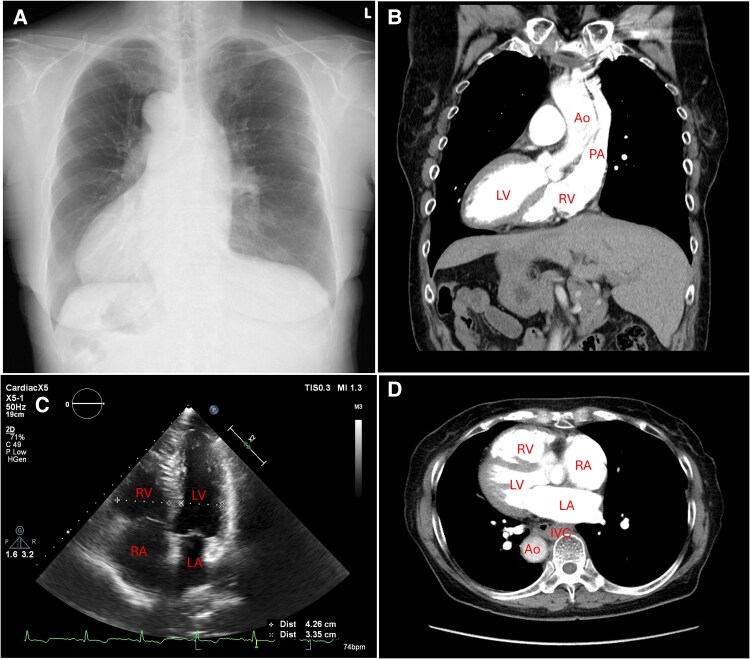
Complete situs inversus on chest X-ray, thoracic echocardiography, and computed tomography. (*A*) Pre-treatment chest X-ray, frontal view. (*B*) Pre-treatment chest computed tomography, coronal view. (*C*) Pre-treatment *trans*-thoracic echocardiogram, four-chamber view. (*D*) Pre-treatment chest computed tomography, axial view. Ao, aorta; IVC, inferior vena cava; LA, left atrium; LV, left ventricle; PA, pulmonary artery; RA, right atrium; RV, right ventricle.
